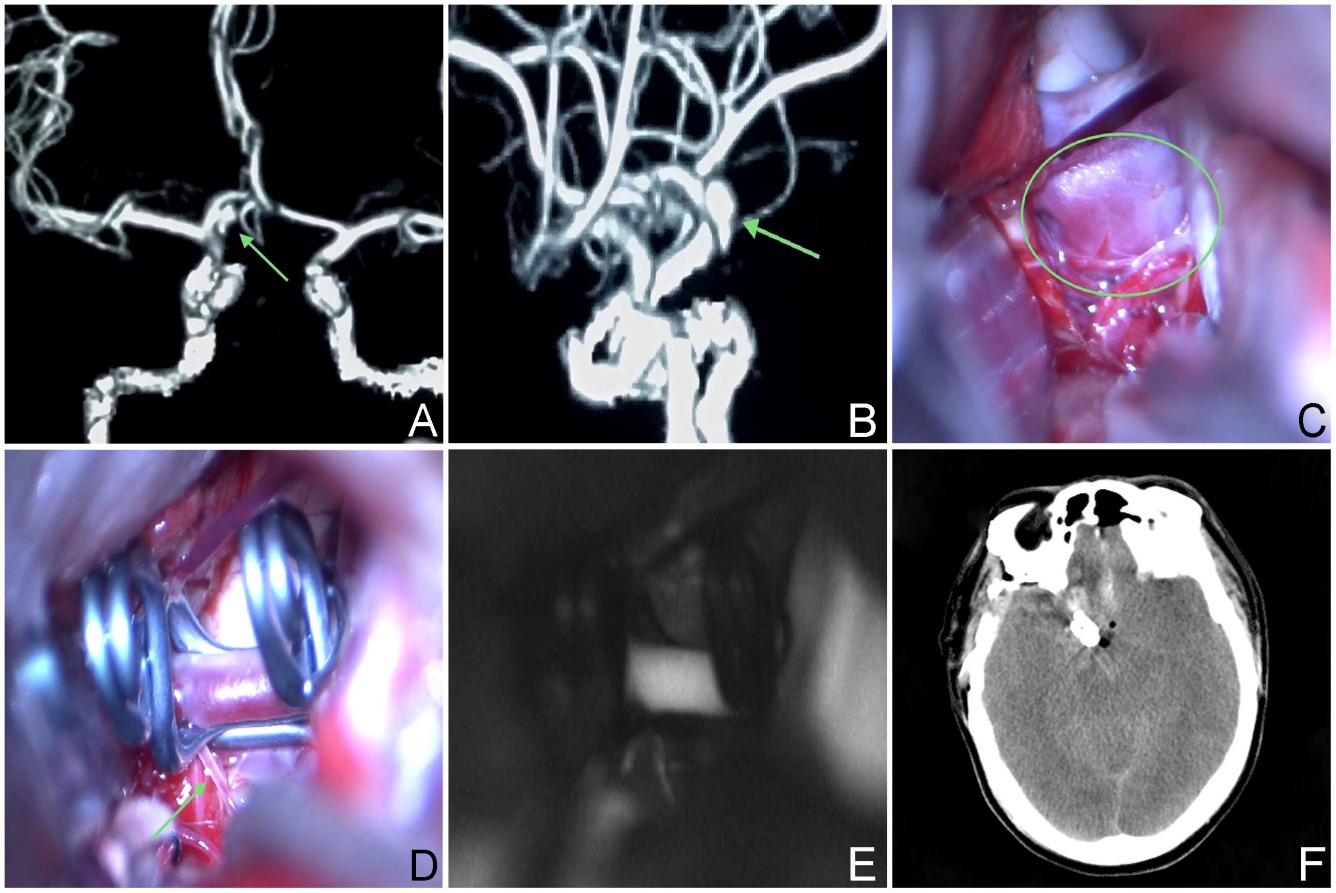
The aneurysm neck is situated within the posterior wall of the parent vessel. (A,B) A 59-year-old male, had an aneurysm that has not ruptured. The CTA revealed that the aneurysm was situated in the posterior wall of the parent artery. (C–E) Intraoperative situation. (F) Postoperative computed tomography scan.
